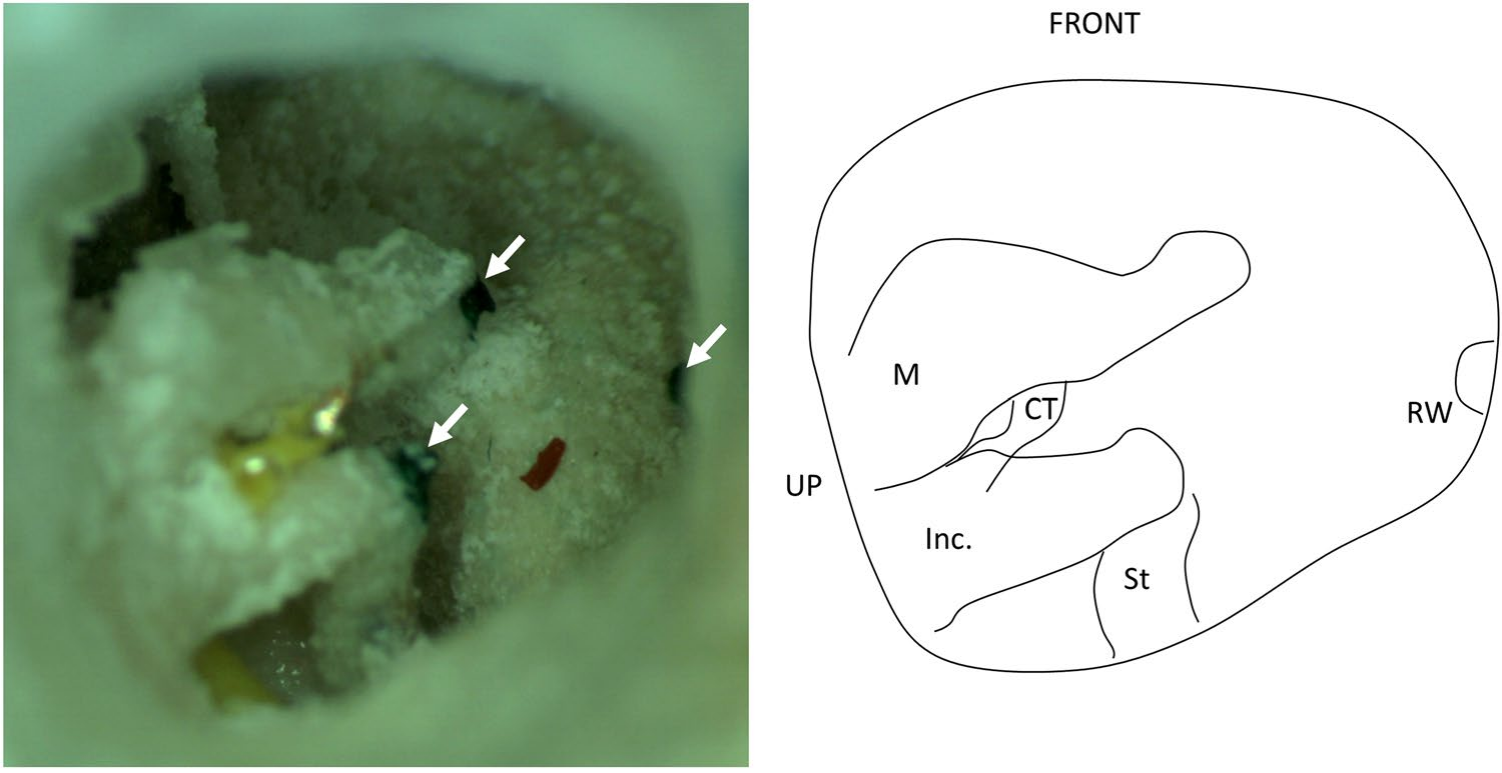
Qualitative analysis of the localisation of the injected droplets. A right temporal bone (TF-ba) is shown in operative position. The black marker dots (arrow heads) represent the targets where the ink droplets were injected. CT: Corda tympani, Inc.: Incus, M: malleus, RW: round window, St: Stapes.
